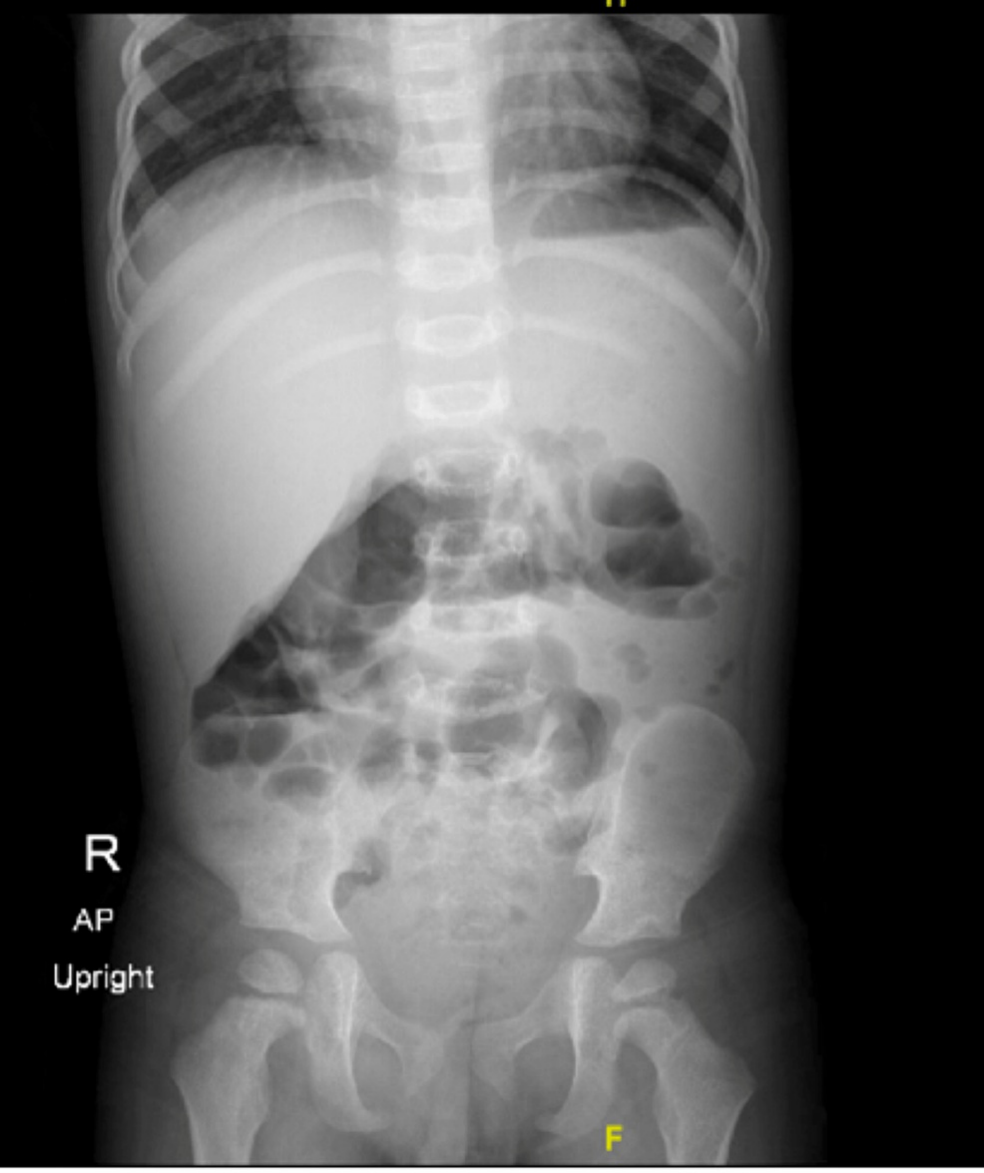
Abdominal X-ray Bowel gas is present in a nonobstructive pattern with no pneumoperitoneum shown.
